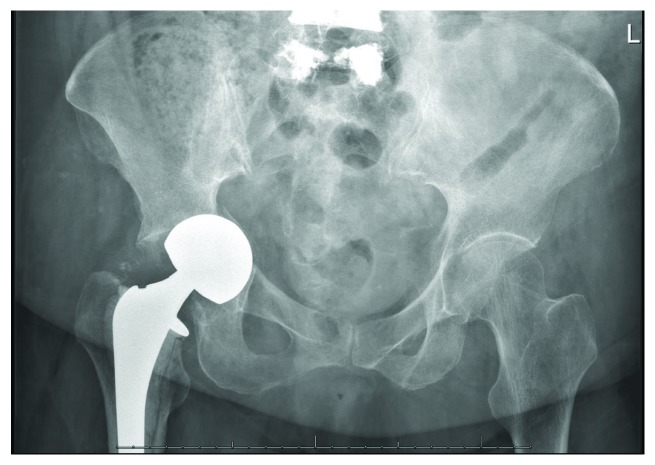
AP pelvis radiograph at 6 weeks postop demonstrating fracture at the medial acetabular wall with superomedial migration of the femoral head, protrusio acetabuli, and no evidence of femoral component loosening.
